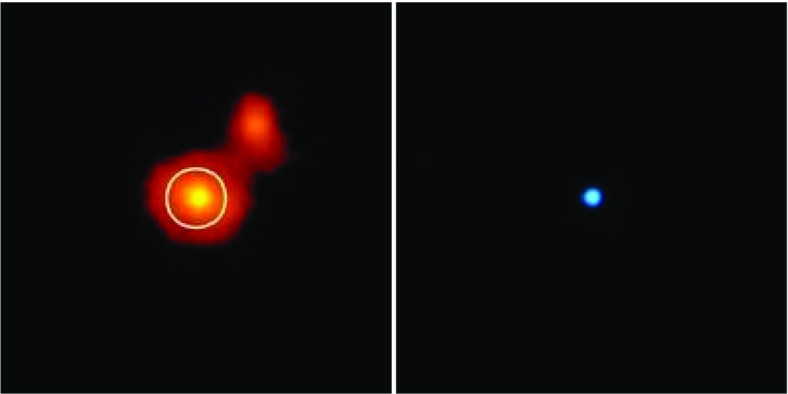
Optical and X-ray images of RX J1242-11. Credits: (left) ESO/MPE/S.Komossa and (right) NASA/CXC/MPE (Komossa et al. 2004)
